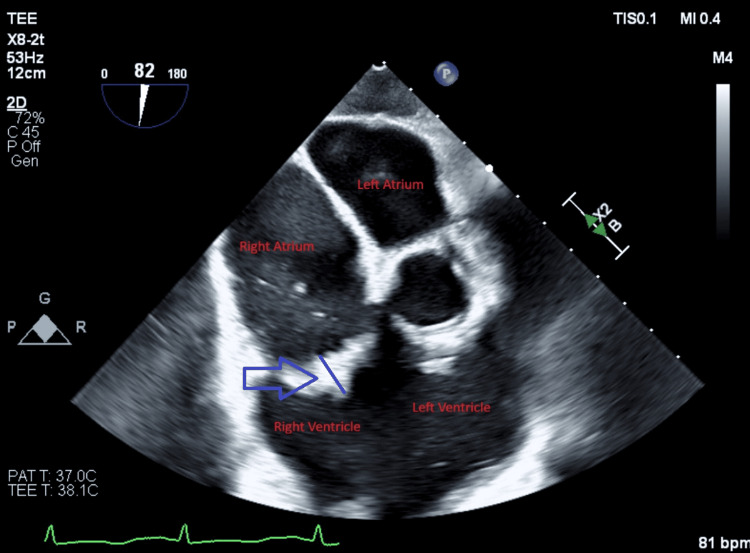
Transesophageal echocardiogram done after AngioVac Blue arrowhead showing decreased vegetations on tricuspid valve
